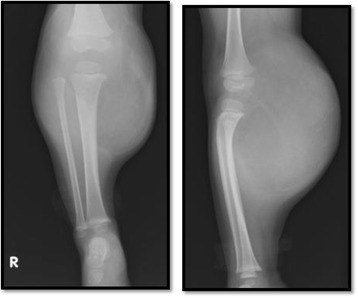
Radiography findings. Anteroposterior (*left*) and lateral (*right*) radiographs showed a localized mass on the popliteal side of lower right leg and proximal tibia deformity
